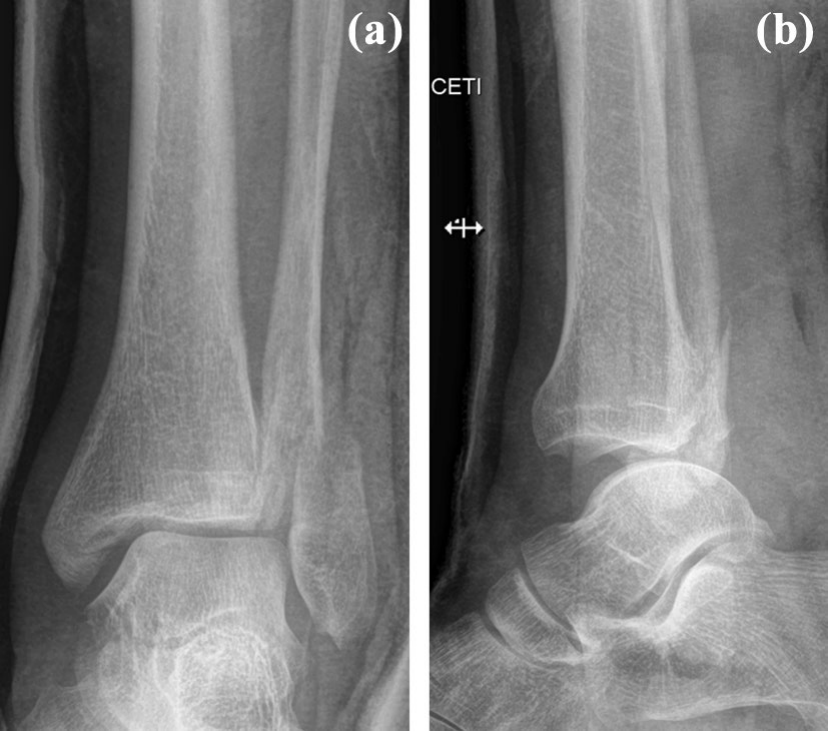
Initial anteroposterior and lateral radiographs with typical signs of a Bosworth fracture-dislocation: a overlap of the distal tibia and fibula, and b posterior dislocation of the talus and tibiofibular dissociation with the fibula being displaced posteriorly
